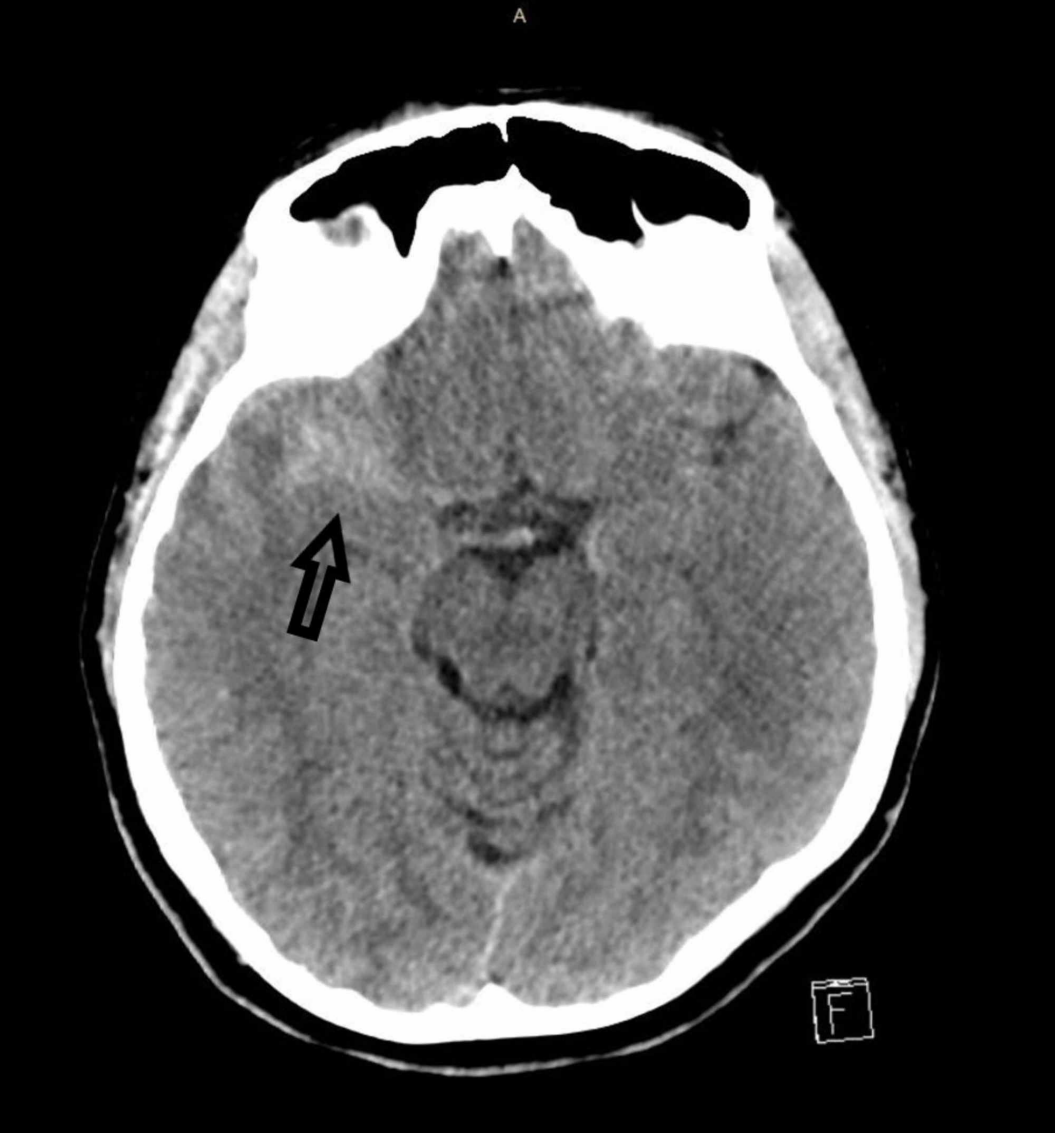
CT of the brain: axial view An axial view of a CT brain showing blood along the right Sylvian fissure.
